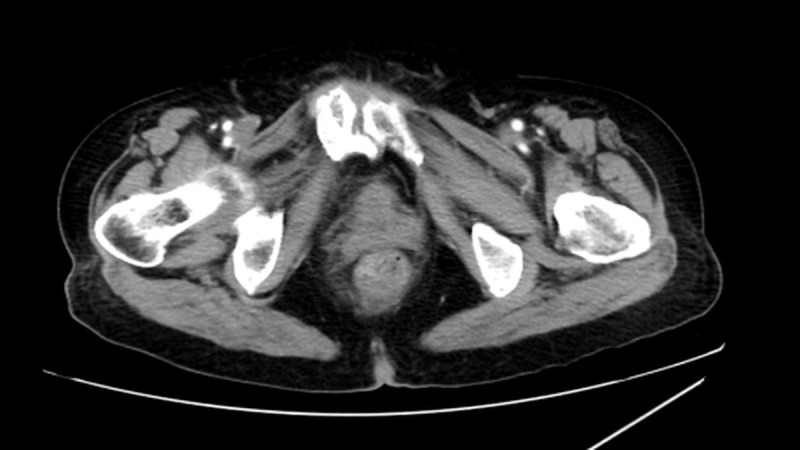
CT imaging characteristics. Distal rectal lesion.
